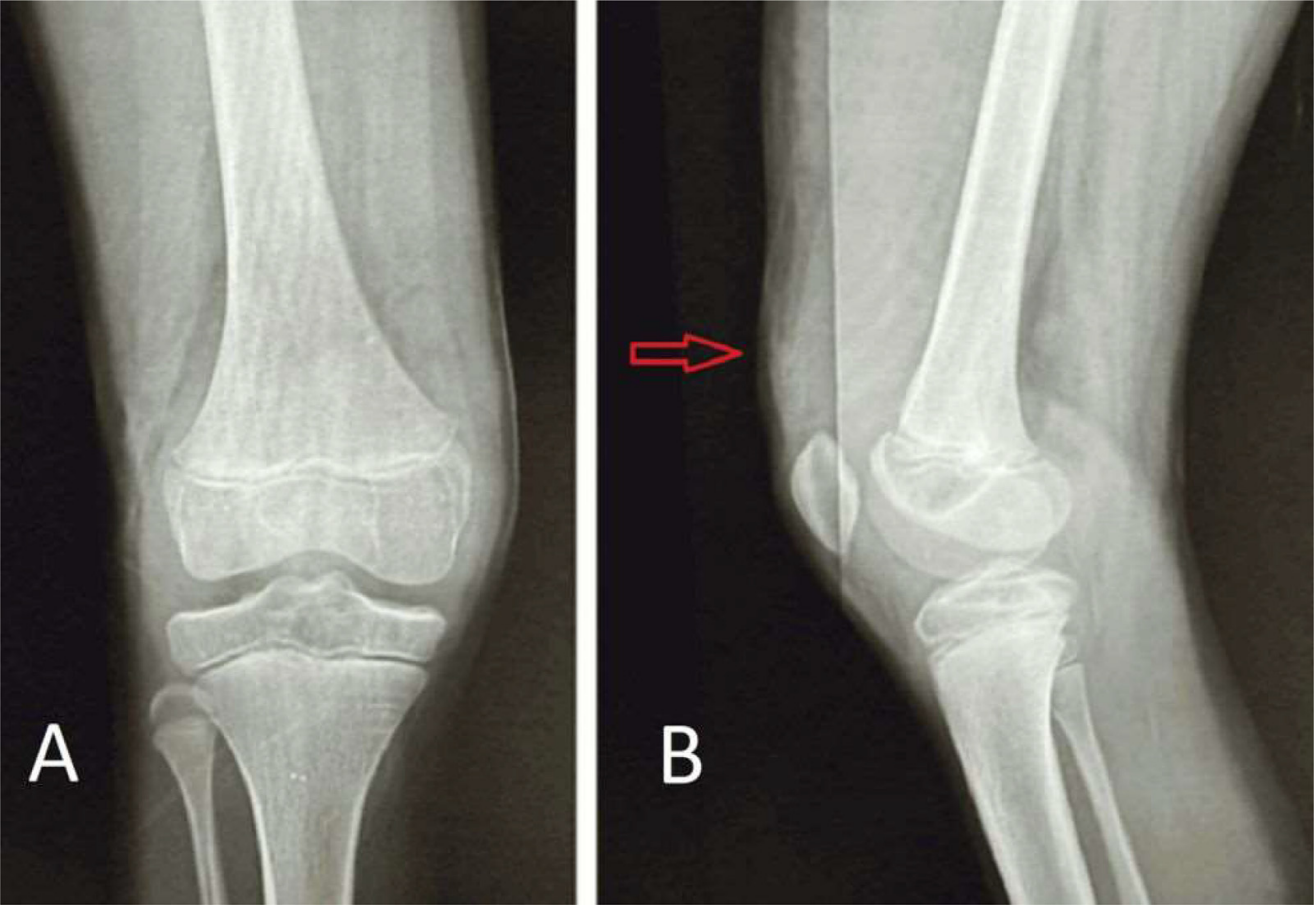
X-ray AP view and Lateral view showing normal knee joint with mild soft tissue swelling on the supra-patellar aspect of right knee (red arrow). A-Xray AP view,B-Xray Lateral view.
